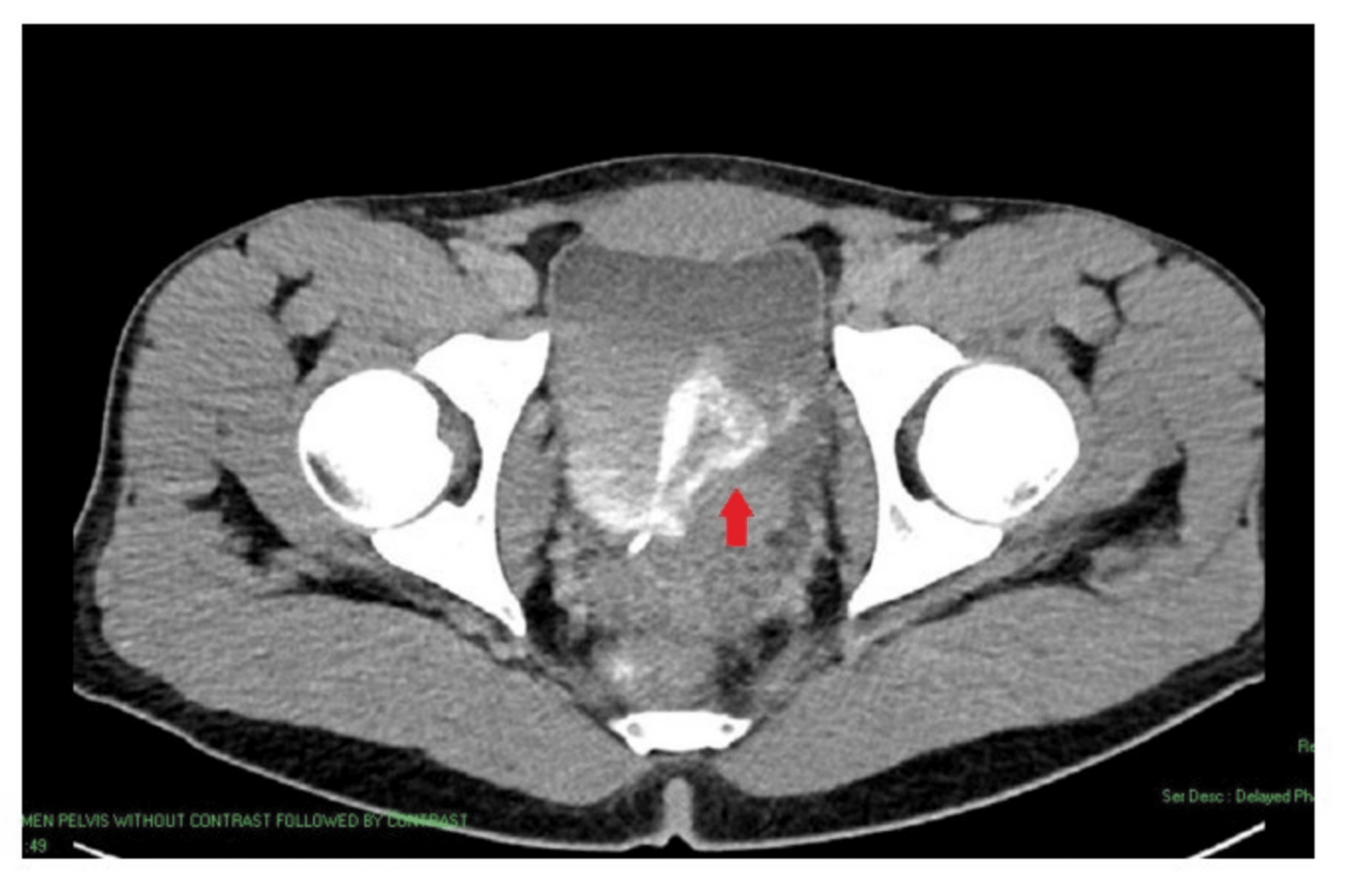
An axial cut of a CT scan taken in the contrast delayed phase. An arrow points to thickening of the left posterolateral aspect of the bladder wall, measuring approximately 4.5 × 4.3 × 3 cm, with internal nodularities observed.
